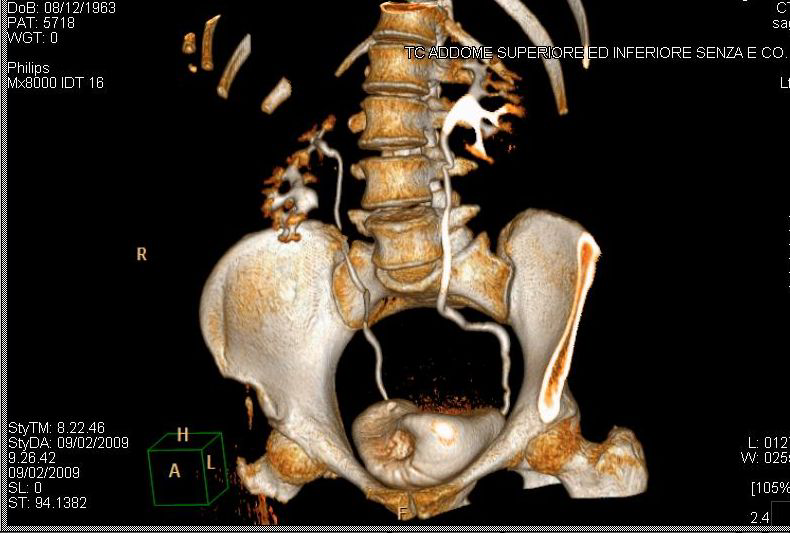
Enhanced abdomen CT scan reconstruction showing the bladder lesion at the level of the anterior wall.
